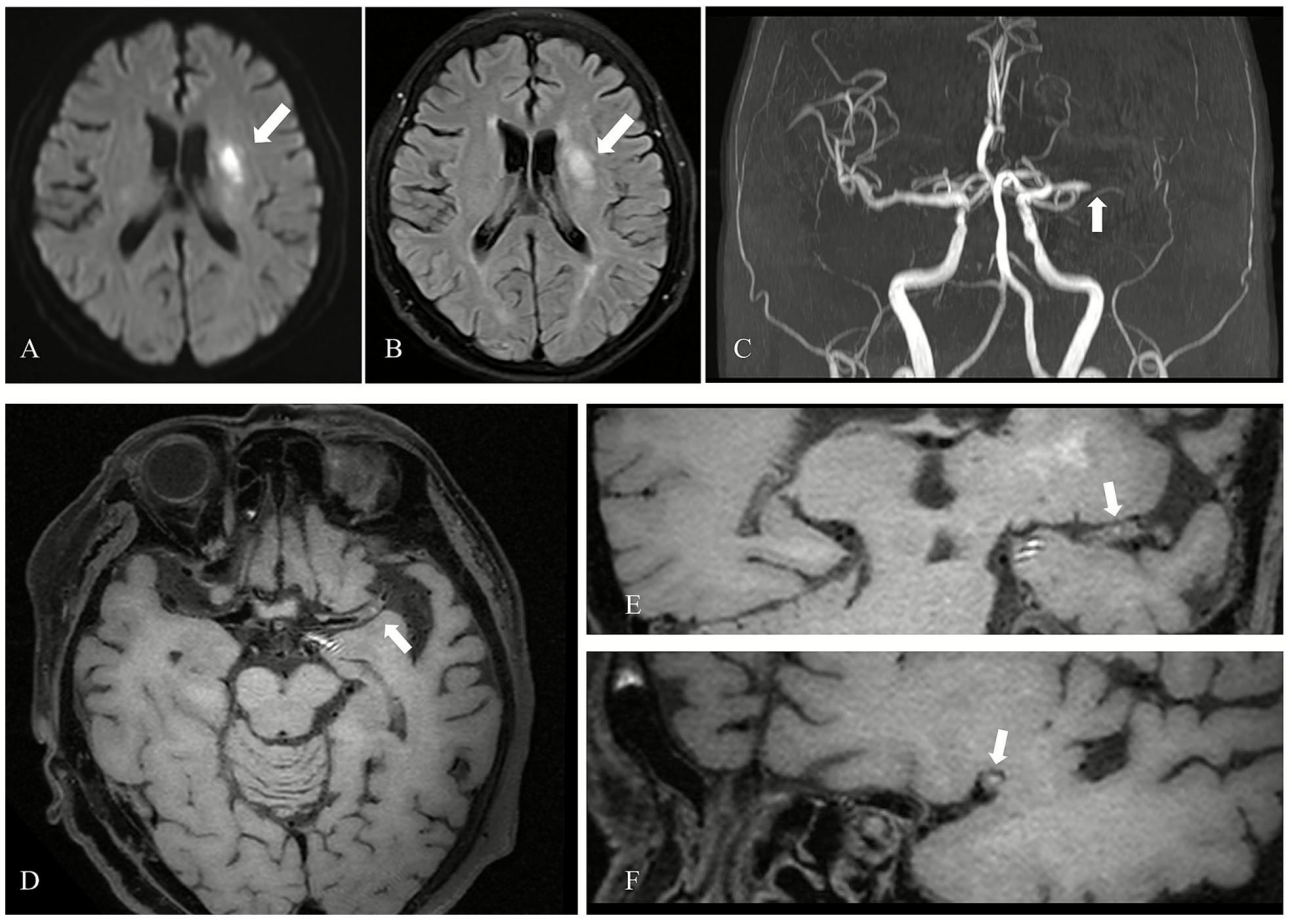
T1-weighted hyperintensity in a culprit plaque in a patient with acute cerebral infarction. (A) DWI shows an acute cerebral infarction in the left basal ganglia (arrow). (B) FLAIR shows hyperintensity at the corresponding region (arrow). (C) MRA shows occlusion in the left MCA (M1 segment) (arrow). (D) Axial 3D-MATRIX T1WI fat-suppressed shows a plaque with punctate hyperintensity (arrow), with signal intensity 1.5 times greater than that of adjacent brain tissue. (E) Coronal image reconstruction perpendicular to the flow direction confirm high signal within the plaque (arrow). (F) Sagittal image reconstruction perpendicular to the flow direction confirms high signal within the plaque (arrow).
